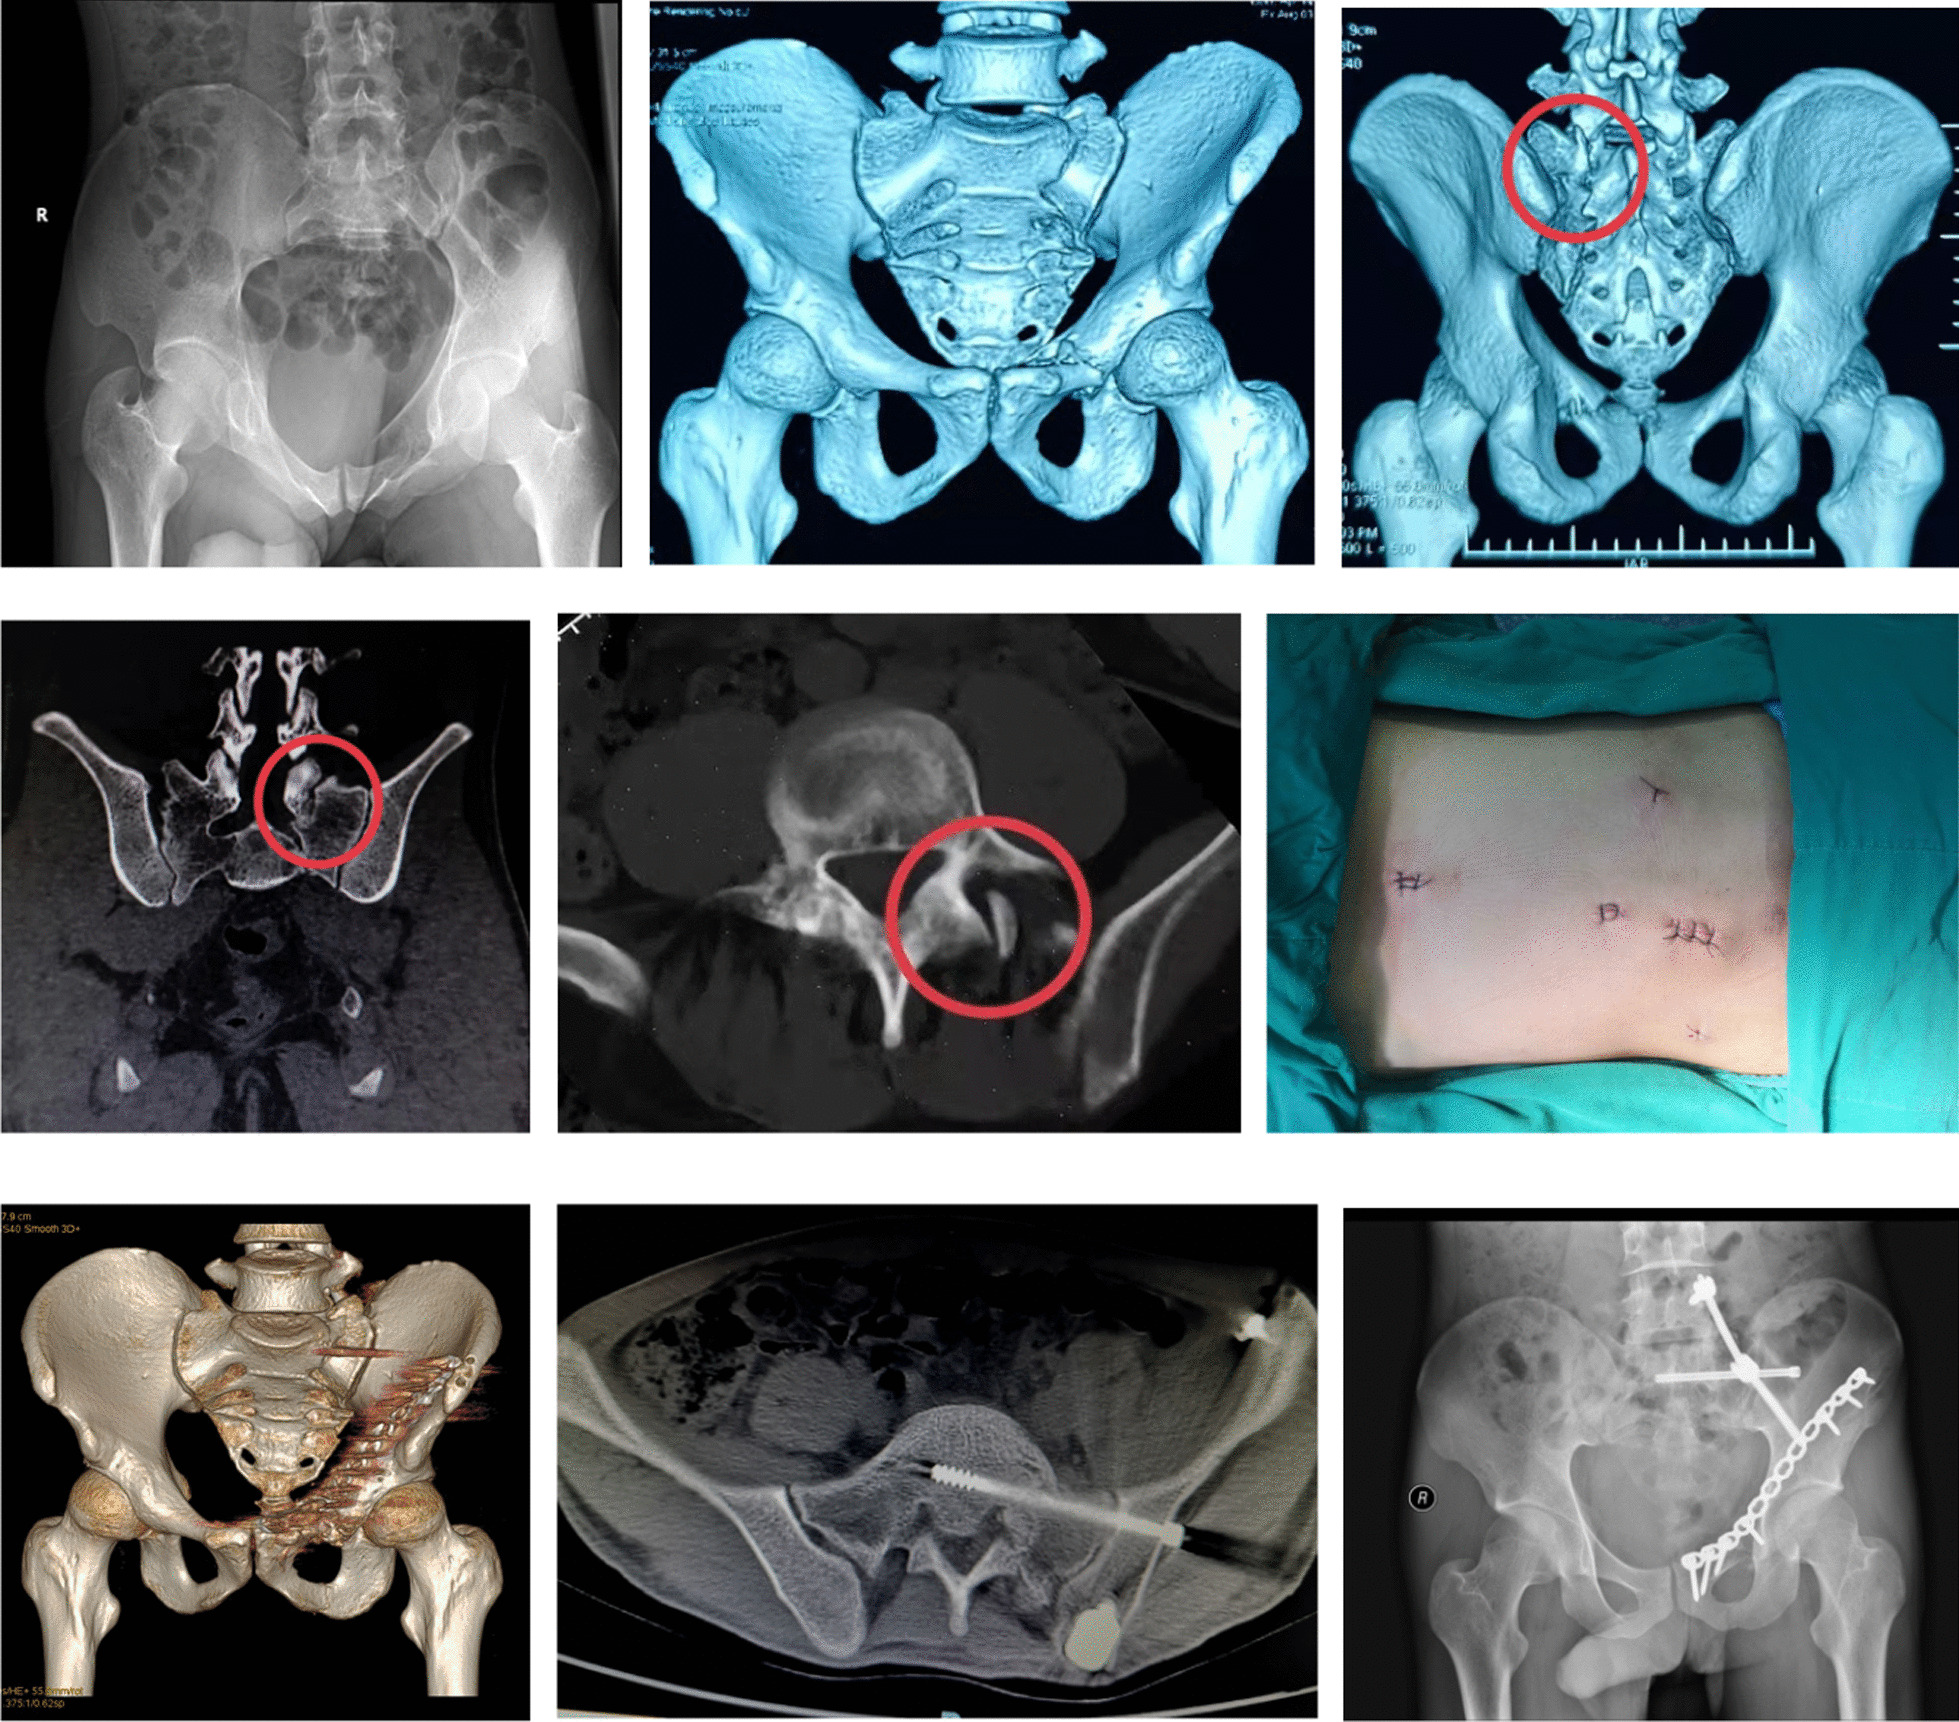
Male, 26-year-old, a crush injury. A. Radiograph on admission showed a displaced pelvic fracture due to the lateral compression mechanism. B The CT reconstruction image revealed an internally rotated displaced fracture on left sacrum combined with ipsilateral superior ramus fracture (Tile type B2). C-E The areas in the red circles on CT views revealed the fracture and subluxation at the level of L5/S1 facet joint (Isler type IIc). F The skin incisions of posterior pelvic ring after minimally invasive TOS with TiRobot assistance. G CT reconstruction taken postoperatively. H Postoperative axial CT view of S1 demonstrated a satisfactory position of the sacroiliac screw. I Postoperative 6-month radiograph showed fractures healing
